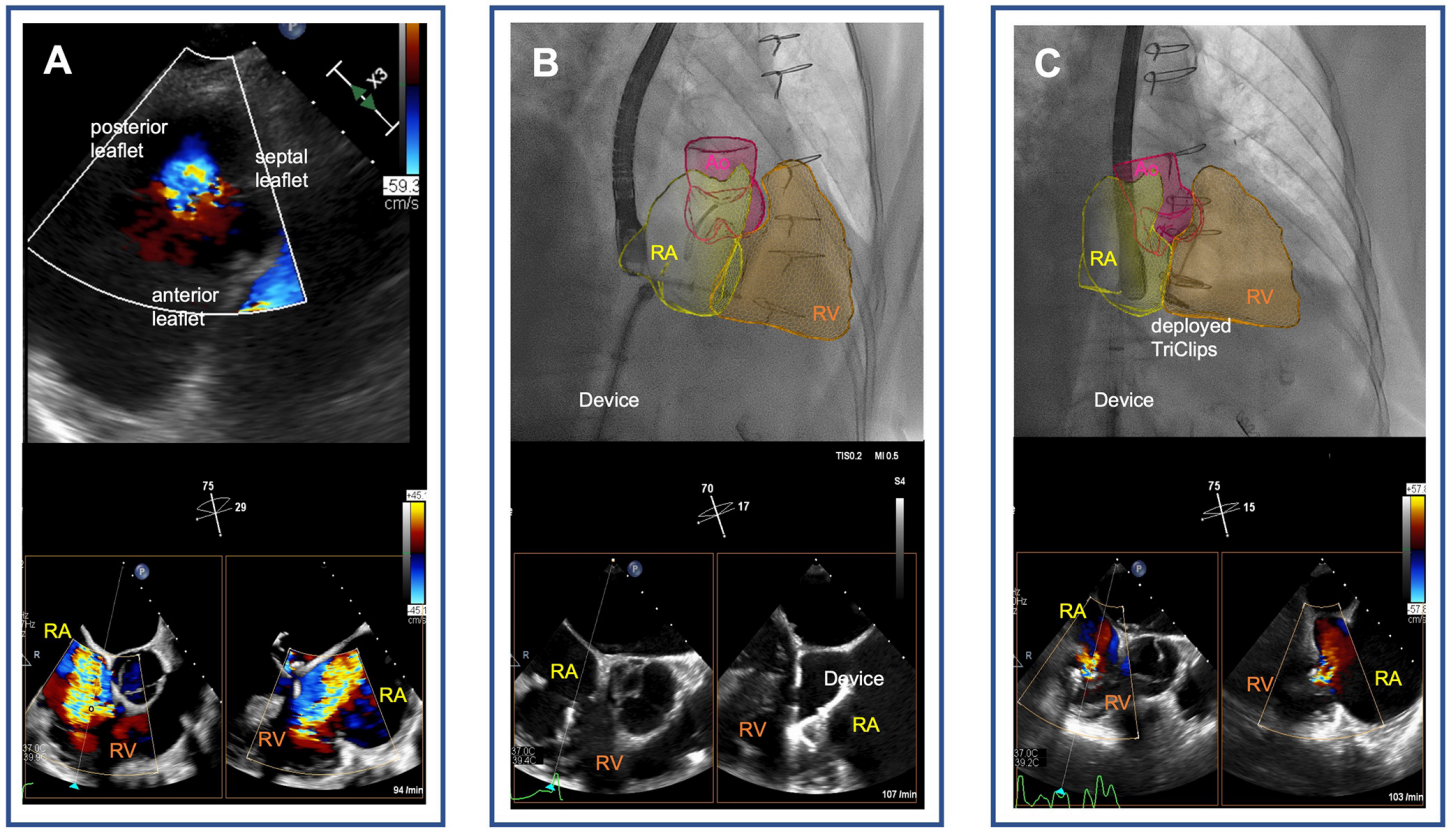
Tricuspid transcatheter edge-to-edge repair following surgical tricuspid annuloplasty. (A) Severe functional tricuspid regurgitation following sutured annuloplasty [transgastric view on the tricuspid valve (upper panel) and biplane transesophageal echocardiographic images of the right ventricular outflow tract (lower panel)]. (B) Real-time fusion imaging-assisted navigation of the transcatheter tricuspid valve repair device through the right atrium pointing to the tricuspid annulus (upper panel). Biplane transesophageal echocardiographic images of the right ventricular outflow tract showing grasping of the septal and anterior leaflets of the tricuspid valve with the TriClip device (lower panel). (C) Mild tricuspid regurgitation after deployment of two TriClips [real-time fusion of echocardiography-derived right heart cavities on fluoroscopy visualizes the TriClips (upper panel), and biplane esophageal echocardiographic image demonstrates reduction of tricuspid regurgitation to mild degree (lower panel)]. RA, right atrium; RV, right ventricle; Ao, aortae.
